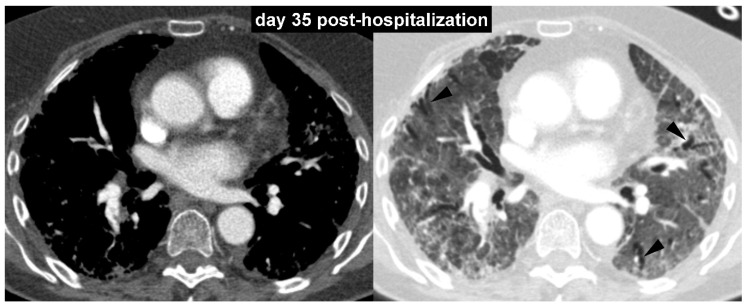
To monitor parenchymal lung disease, serial chest radiographs with different time intervals were obtained. However, to evaluate the response of pulmonary vascular disease to anticoagulant therapy, a further contrast-enhanced CT scan was performed after one month (day 35 post-hospitalization). The follow-up contrast-enhanced CT images with mediastinal and lung window setting (Figure 2) showed significant regression of the pulmonary embolism and lung abnormalities (both consolidations and ground-glass opacities). However, initial signs of lung architectural distortion with some traction bronchiectasis (arrowheads) were observed.
